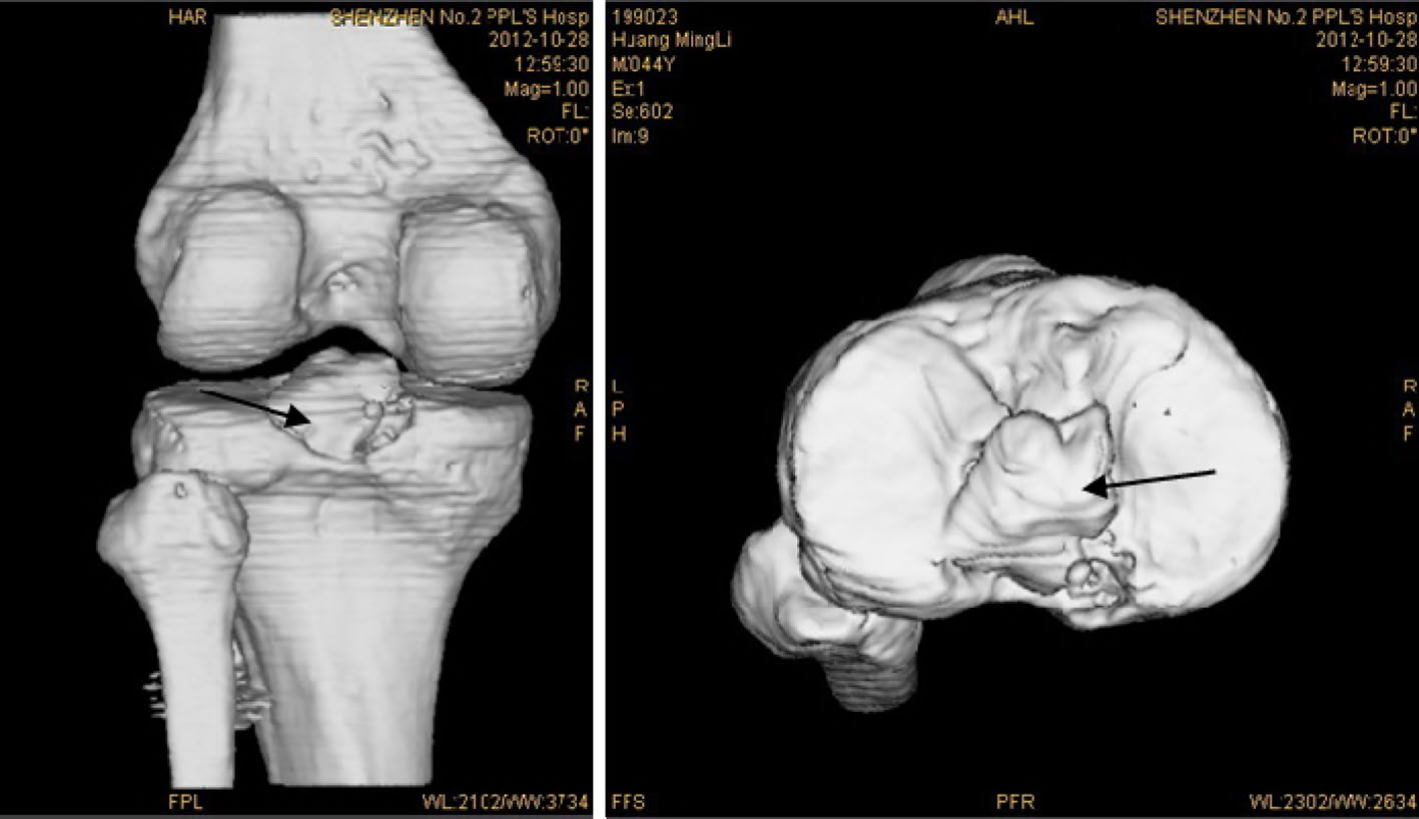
Postoperative three-dimensional reconstructed CT scan. Postoperative three-dimensional reconstructed CT scan shows good fixation and reduction at the distal insertion of the posterior cruciate ligament (represented by the *arrow*)
